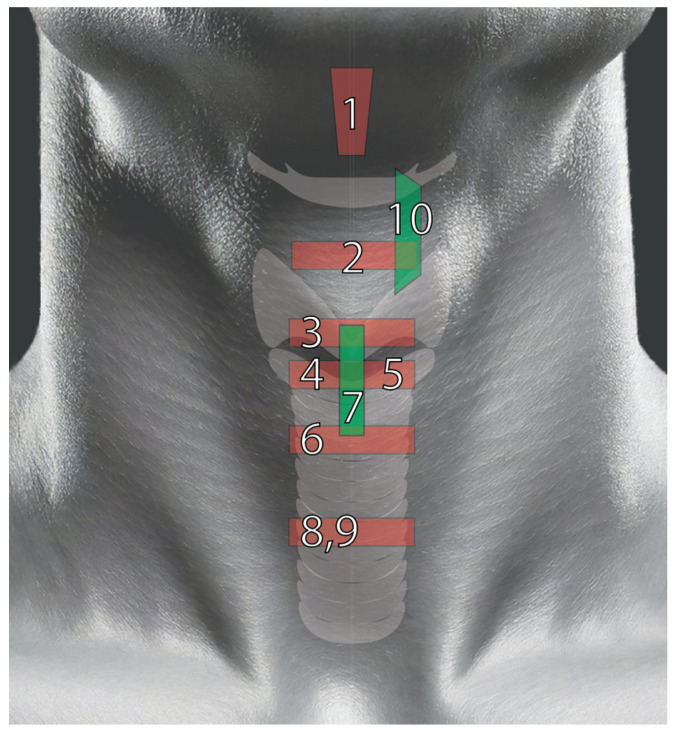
Airway ultrasound probe positions. The red boxes represent the approximate location of the footprint of the probe on the surface of the skin and its orientation (longitudinal or transverse). All views are performed with the use of a high-frequency linear probe, with the exception of the suprahyoid view. 1, Suprahyoid view, performed with a curved linear probe; 2, Thyrohyoid view; 3, Thyroid view; 4, Transverse cricothyroid membrane view; 5, Suprasternal view of the cricoid; 6, Suprasternal view of the tracheal rings; 7, Suprasternal longitudinal view; 8, Suprasternal view to identify the esophagus; 9, Suprasternal view to identify endotracheal tube; 10, Parasagital Thyrohyoid view.
